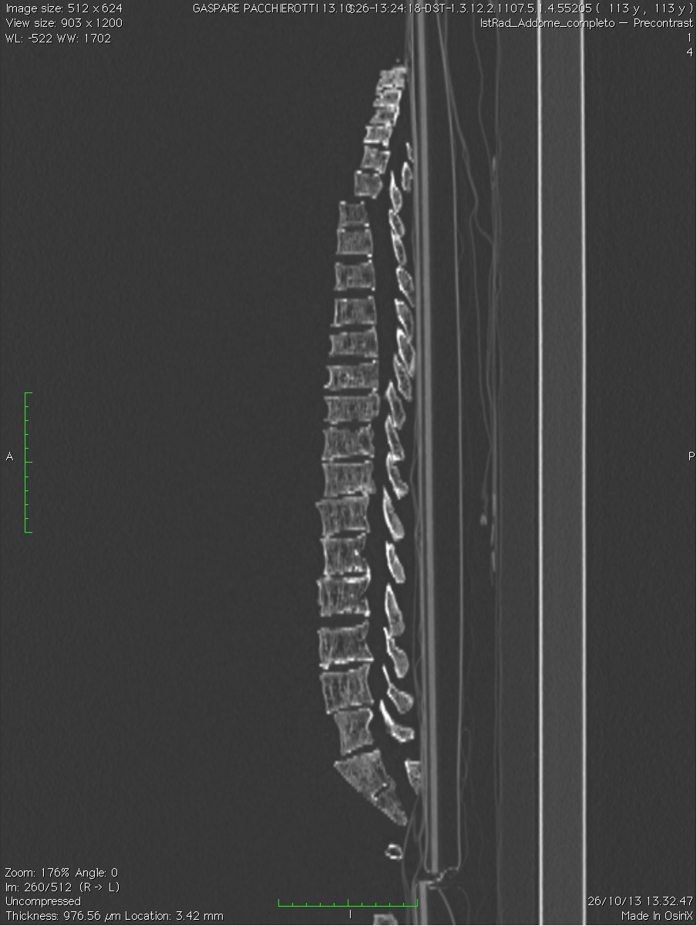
CT scan of the spine of Pacchierotti.
